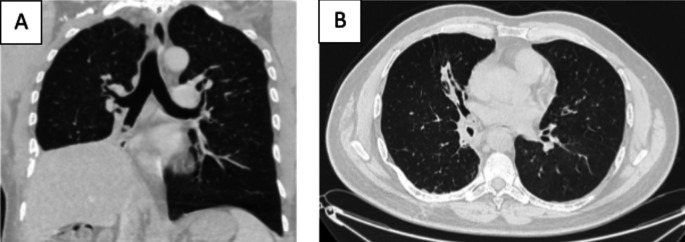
Chest CT-scan (A-B): the coronal and axial views: Endobronchial lesion of fatty density in the middle lobe bronchus, responsible for minimal bronchiectasis.
